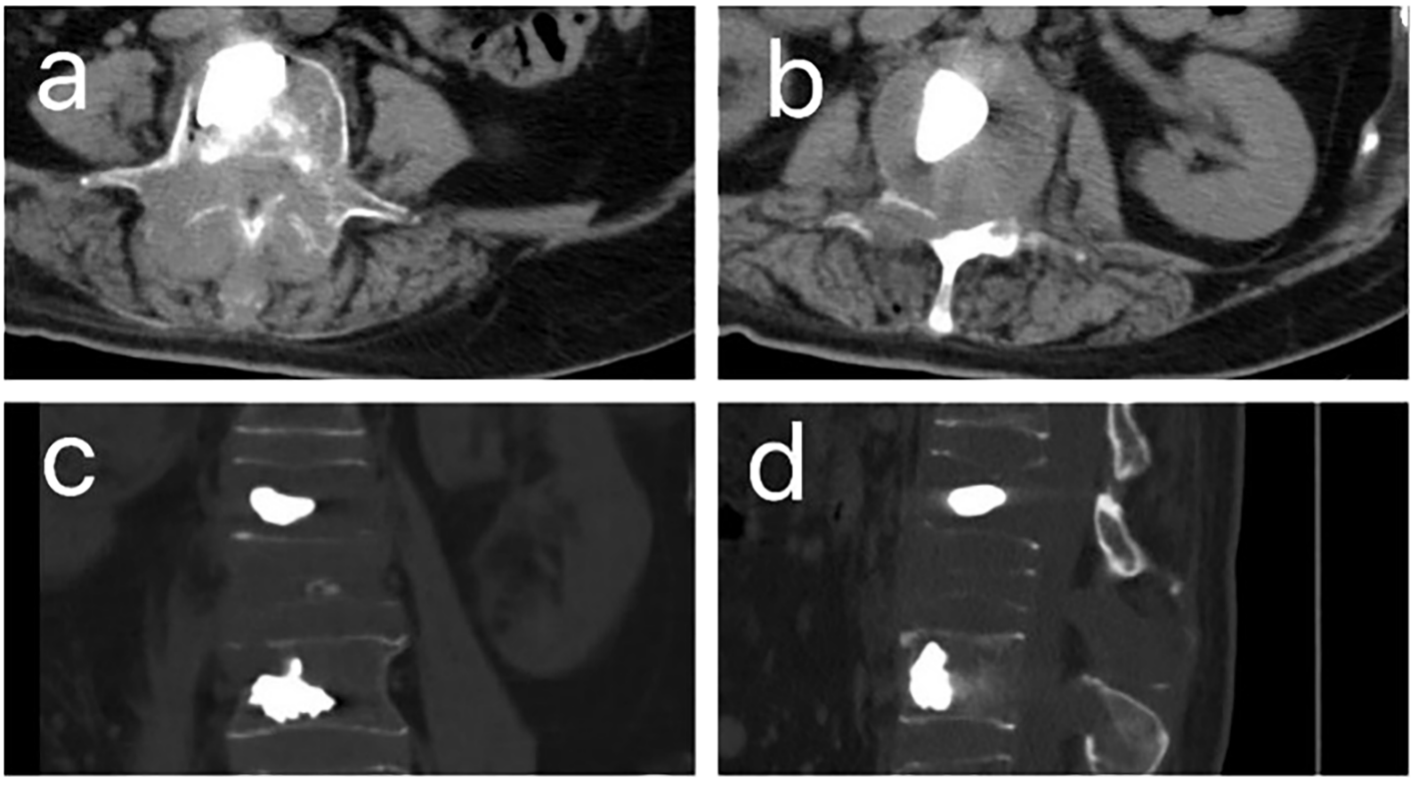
CT images after percutaneous kyphoplasty (PKP) of patient. (A, B). The horizontal plane CT image of patient after PKP. (C). The coronal CT images of patient after PKP. (D) The sagittal CT images of patient after PKP.
